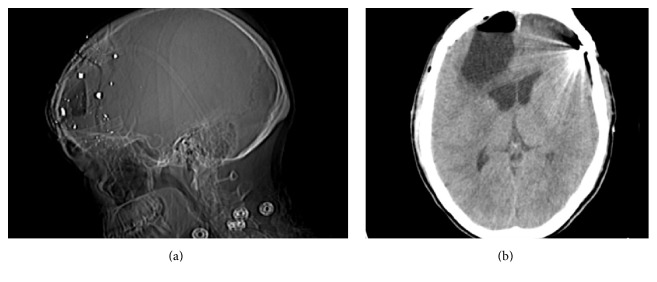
Postcranioplasty CT head. (a) Scout image showing reduction in size of pneumocephalus. (b) Axial noncontrast head CT demonstrates marked resolution of pneumocephalus and decreased midline shift and mass effect on the right lateral ventricle.
